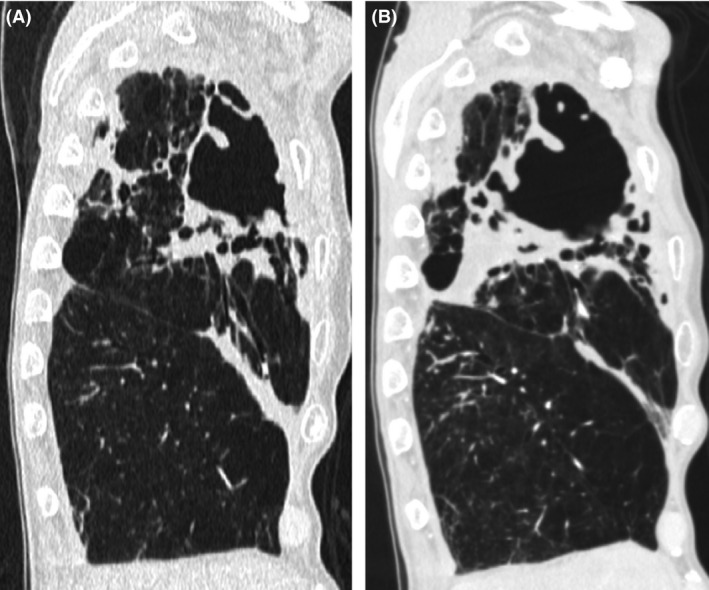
(A) Computed tomography image showing consolidation and large fibrocavitary changes in the right upper lobe. (B) Computed tomography image obtained four months later showing progression of consolidation and fibrocavitary changes.
